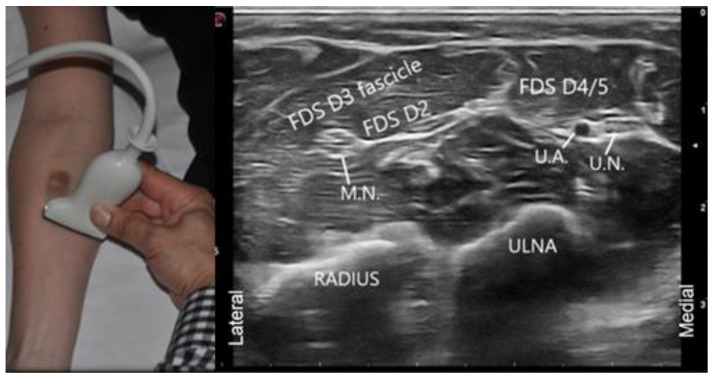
Notch positioning. When injecting the right upper limb, we suggest that the notch be placed laterally as opposed to the radiologic convention of notch medial. The position of the notch laterally allows for the ultrasound image to be a direct reflection of the movement of the ultrasound probe on the skin. (FDS: Flexor Digitorum Superficialis/Sublimis, M.N.: Median Nerve, U.A. Ulnar Artery, U.N.: Ulnar Nerve) (Images acquired on Esaote MyLab 7 ultrasound machine, Esaote ultrasound machine with 13–3 Hz linear array transducer, (Esaote; location: Fishers, IN, USA)).
